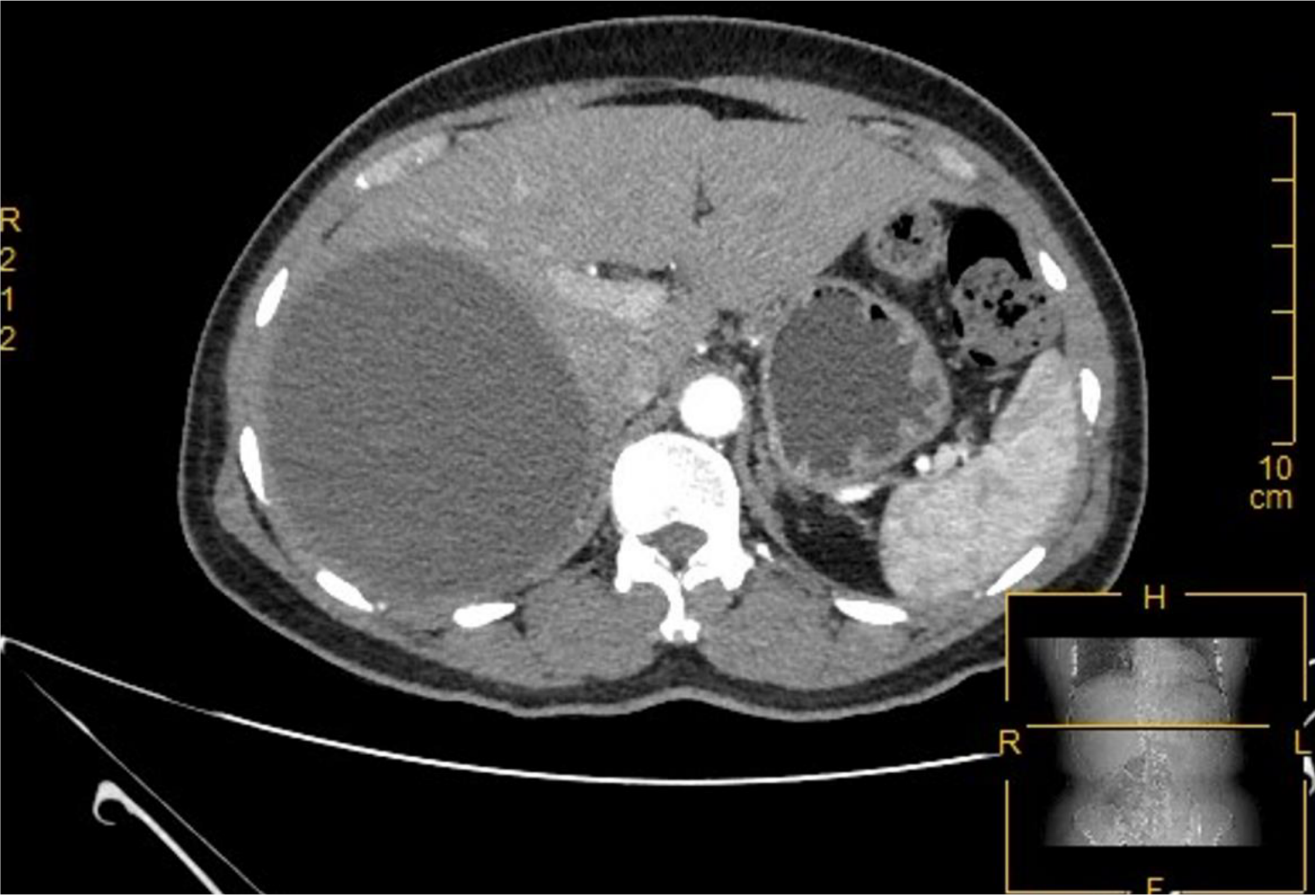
Arterial phase of enhanced CT: This was a large, round, hypodense lesion located in the right posterior lobe of the liver, measuring 10.8 cm × 9.5 cm.
